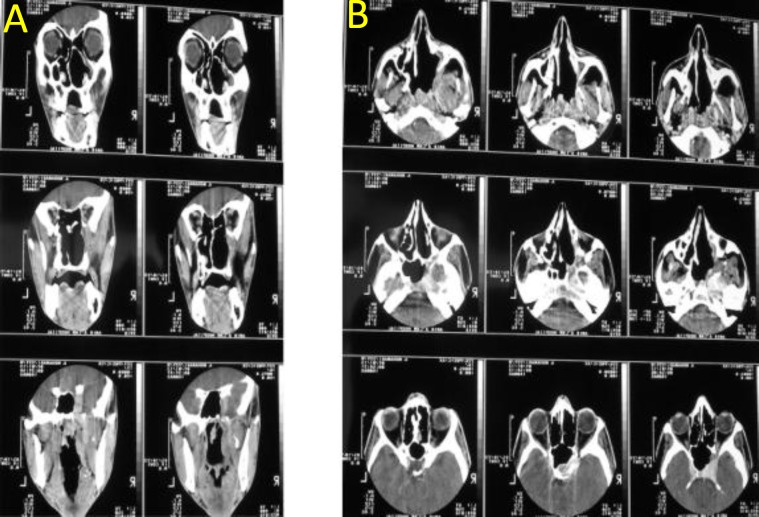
Postoperative coronal (A) and axial (B) views of contrast CT scan of the patient six months after tumor removal via exclusively endoscopic transnasal surgery
